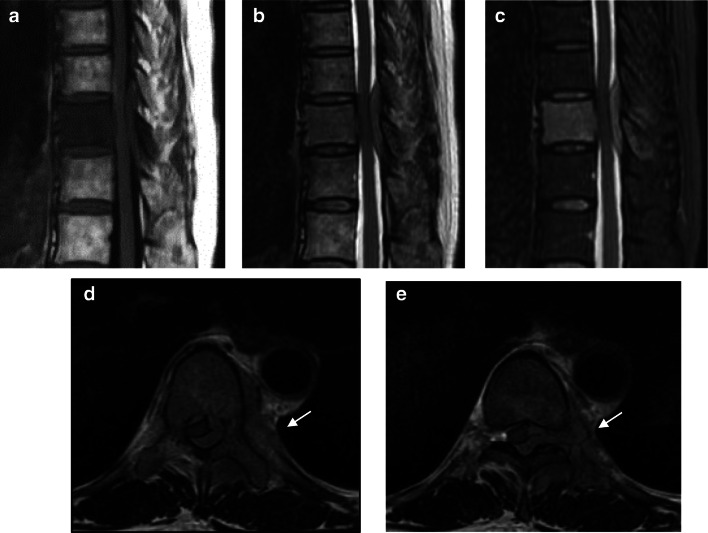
Magnetic resonance imaging of the thoracic spine. (a–c) Sagittal image of T1- and T2-weighted images, and short-tau inversion recovery images. (d, e) Axial image of T2-weighted images. The lesion on the lateral side of the Th10 vertebral body showed a low signal intensity on the T1- and T2-weighted images and high signal intensity on the short-tau inversion recovery images (*white arrow*). The signal change extended through the pedicle to the posterior elements, including spinous process. Soft-tissue lesion extended to the epidural space, intervertebral foramen, mediastinal area, intercostal space, and paravertebral muscle, showing the same signal intensity as the bone lesion. The epidural lesion compressed the spinal cord. The nodules reminiscent of lymph nodes were revealed on the dorsal side of the aorta. These findings suggest that the vertebral lesion should extend to the epidural space and paravertebral mediastinum and surrounded the muscle without bone destruction
